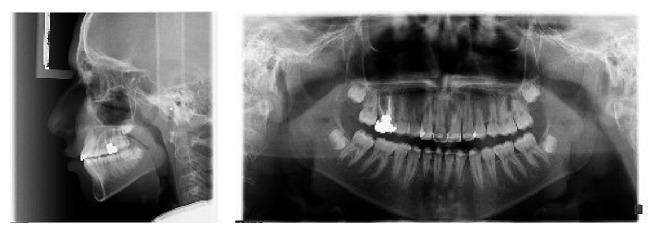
Posttreatment lateral cephalometry and panoramic radiographs.
